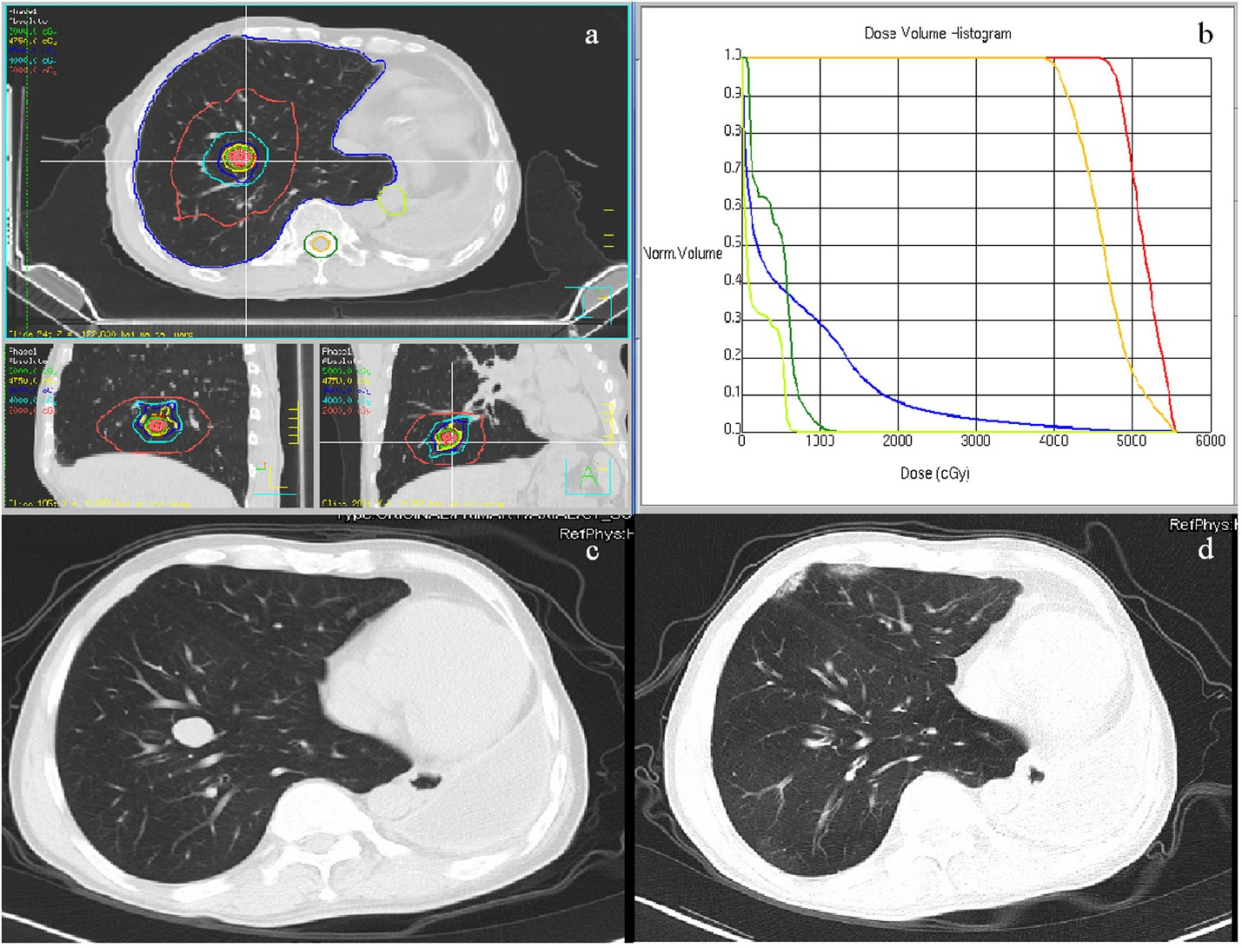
Complete response after SBRT in the representative patient. (a: irradiation isodose curves of the SBRT plan 50 Gy in 5 fractions; b: dose-volume histogram of the SBRT plan; c: CT image before SBRT; d: CT image 9 months after SBRT).
